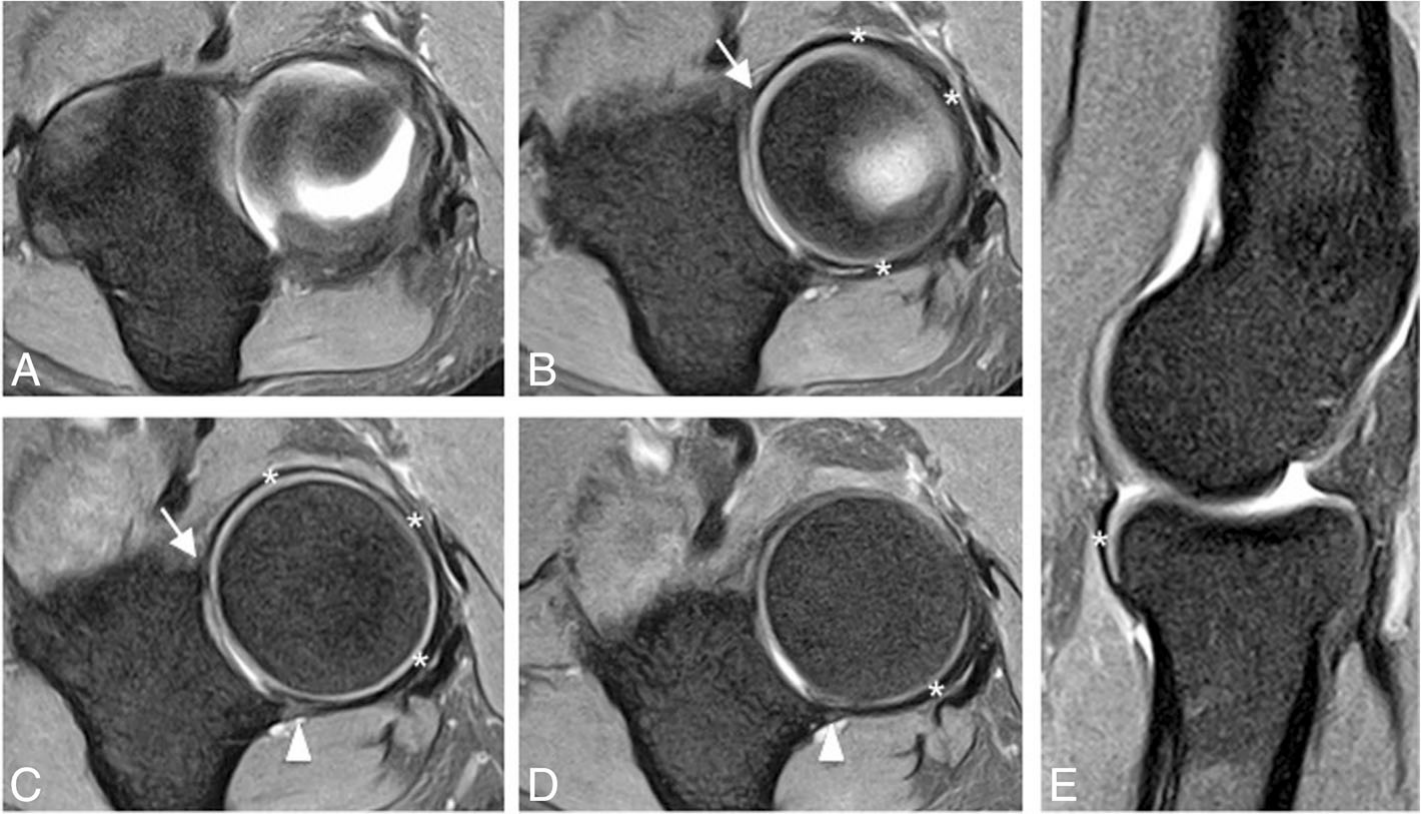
Axial FS PD-weighted MRI (a–d) and sagittal FS PD-weighted MRI (e) showing the intact annular ligament (white asterisks). Anterior attachment of the annular ligament (white arrow). The posterior attachment of the annular ligament can be fenestrated (white arrowheads). The annular ligament encircles the periphery of the radial head (white asterisks in b-d)
